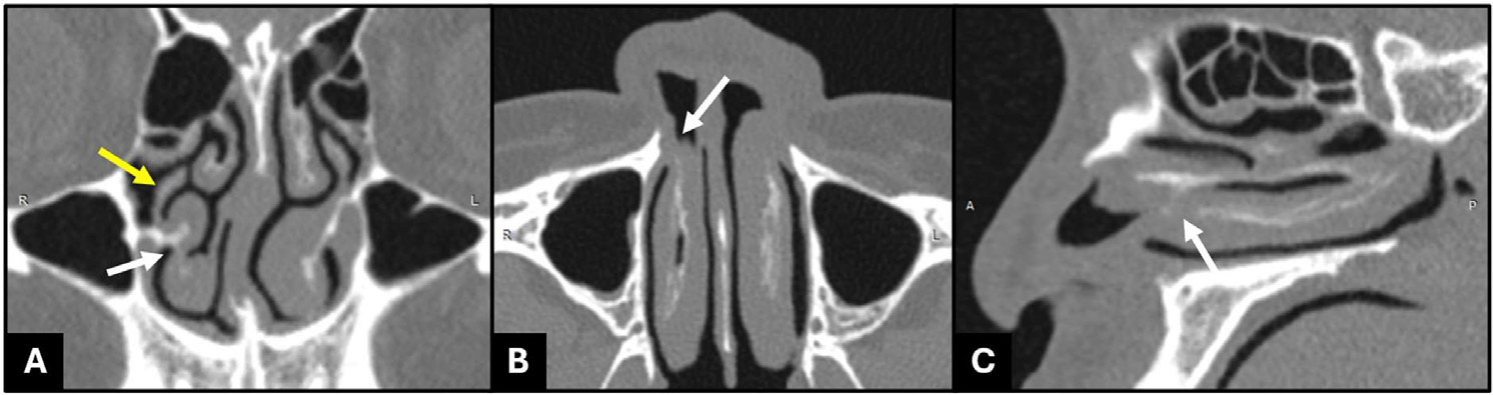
Coronal (A), axial (B), and sagittal (C) paranasal sinus CT depicted 2 inferior turbinates at the right nasal cavity that have the same root, indicating a bifid inferior turbinate (white arrow). The ipsilateral uncinate process (yellow arrow) is present.
